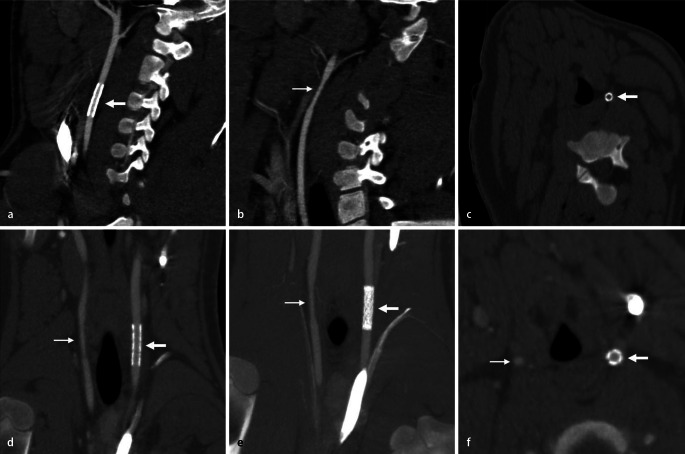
Representative CT images of the common carotid artery, fitted with both types of stent, in the sagittal (a, b), coronal (d, e) and axial (c, f) planes. c Without contrast medium. e Multiplanar reconstruction. *Thin arrows* point to a PLLA/P4HB stent, *thick arrows* to a 316L stent
